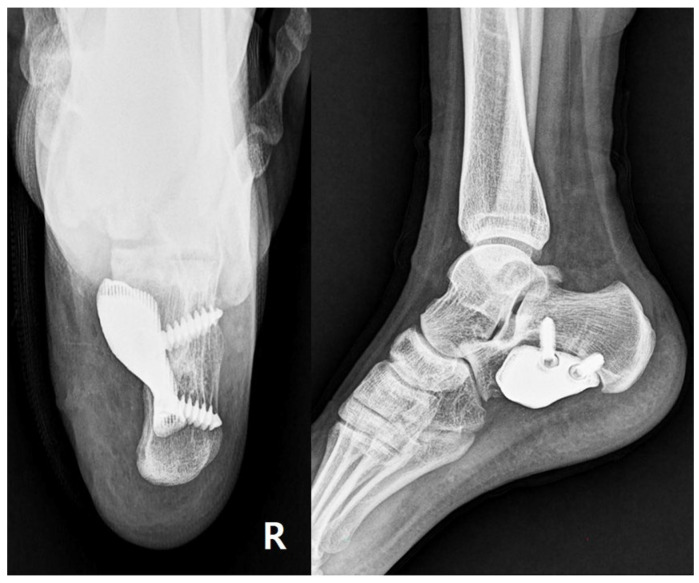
Postoperative imaging. Postoperative radiographs of the calcaneus of right foot in axial and lateral views showing stable fixation and prosthesis placement.
